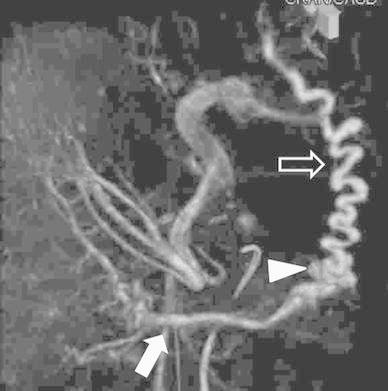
Superior mesenteric arteriography (cone beam CT, venous phase) demonstrates mesenteric varices (*arrowhead*) from the jejunal vein (*arrow*) to a draining vein in the abdominal wall (*open arrow*)
